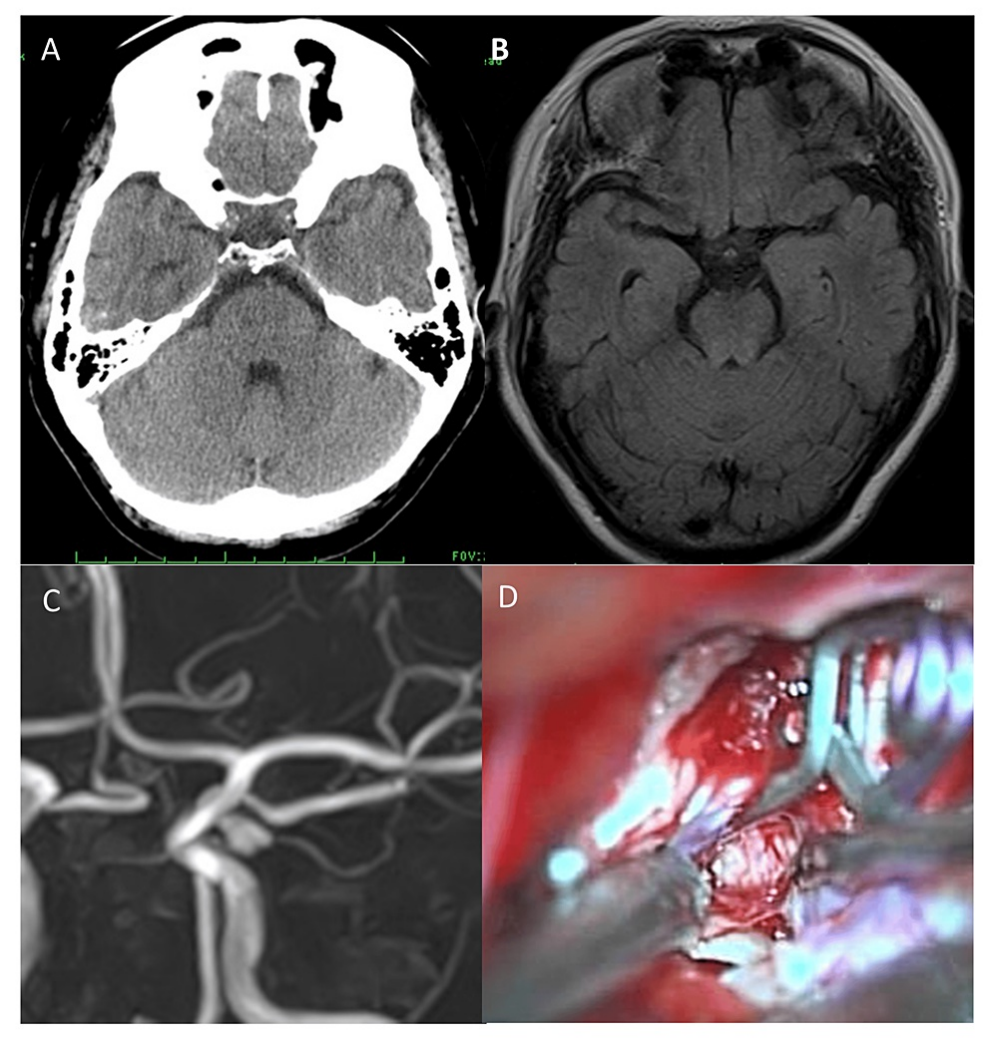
Case 1 Computed tomography (CT, 1A) and fluid-inversion attenuated recovery (FLAIR)-MRI (1B) scans at the initial examination. No SAH was detected. MR angiography (MRA) revealed the inferolateral-directed ICA-PcomA aneurysm with bleb (1C). Intraoperative finding (1D) revealed that the aneurysm firmly adhered at a site behind the anterior clinoid ligament. Partial resection of the anterior petroclinoid ligament was required. SAH: subarachnoid hemorrhage
